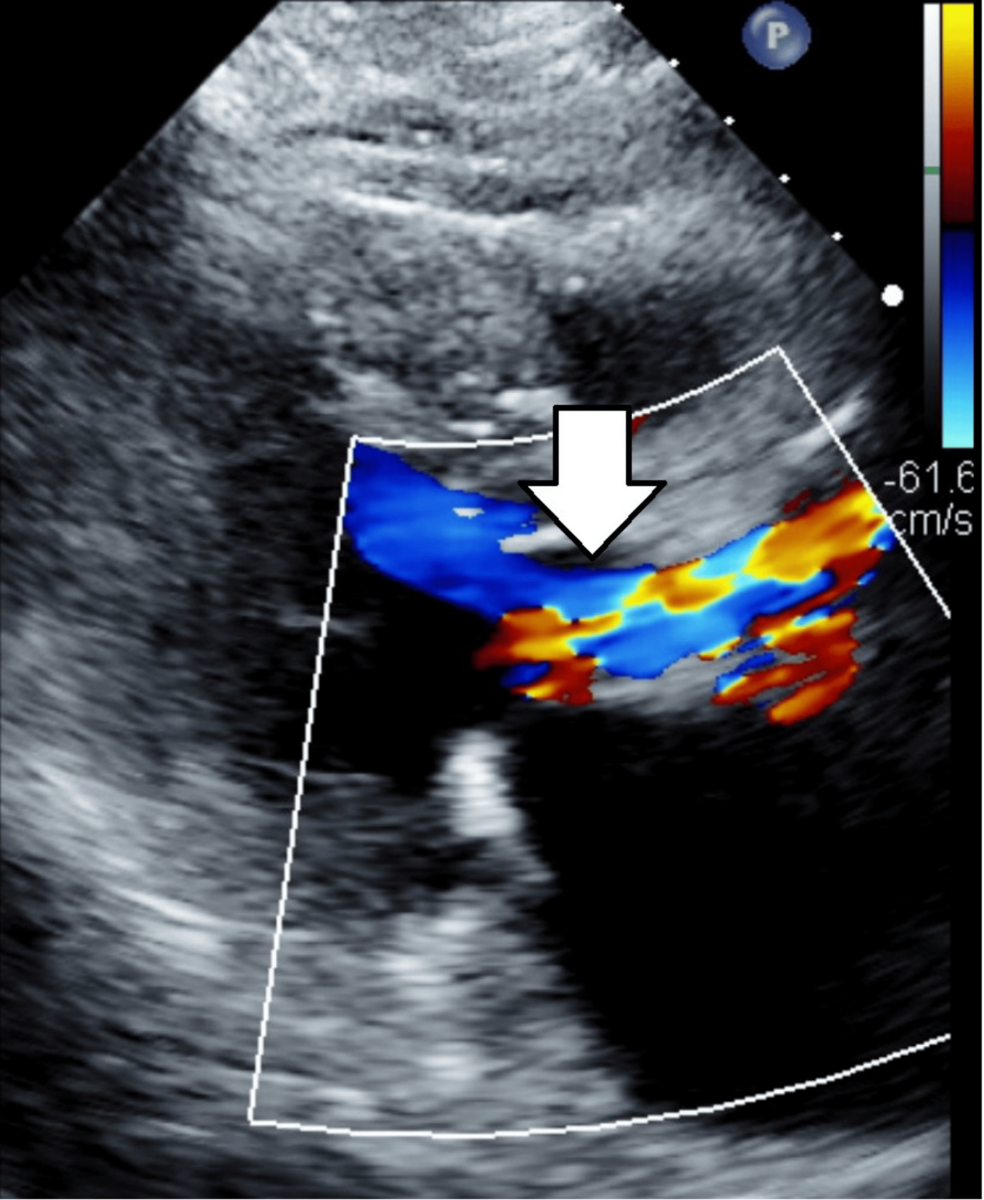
Pre-procedure Transthoracic Echocardiogram Low-flow, low-gradient aortic stenosis, as indicated by the arrow. Aortic valve area (AVA) = 0.7 cm^2^, mean gradient = 36 mmHg, aortic velocity = 3.0 m/sec, left ventricular outflow tract to aortic valve (LVOT/AV) ratio = 0.26, left ventricular ejection fraction (LVEF) = 45%, and transvalvular stroke volume index = 32 mL/m^2^.
